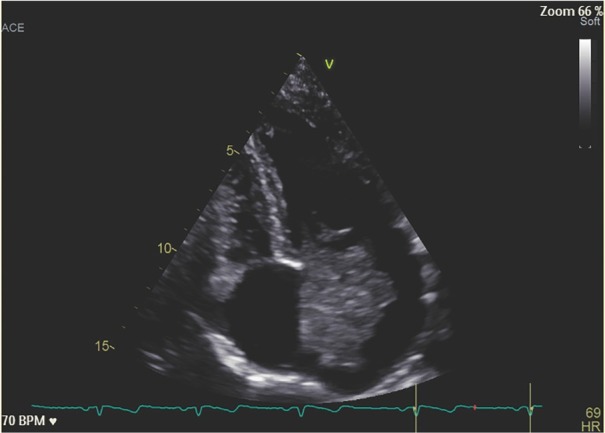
Echocardiographic image of the AM occupying almost all the left atrium and mitral valve. AM, atrial myxoma.
